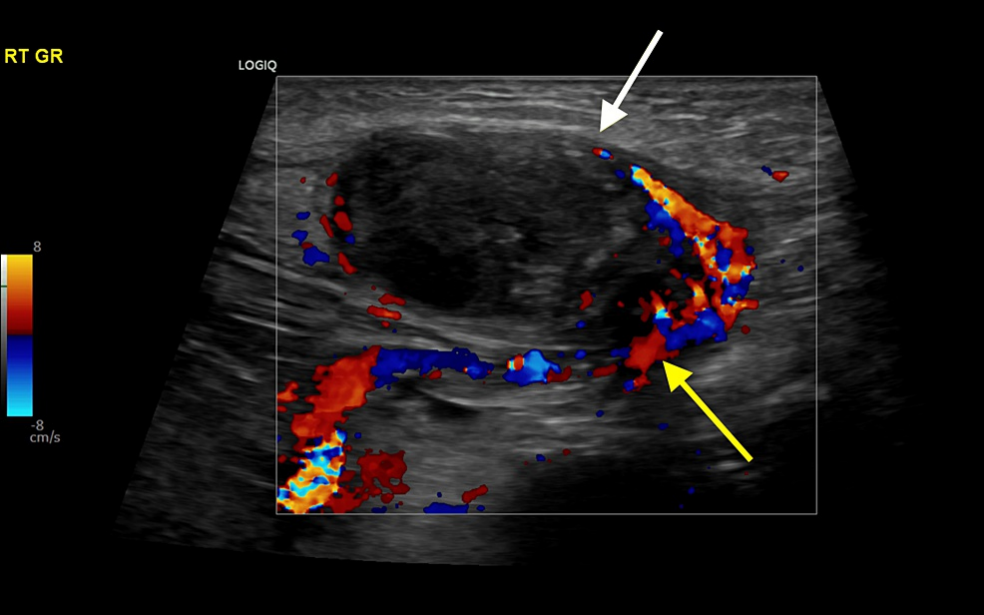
A color doppler ultrasound image of the right groin shows a heterogenous hypoechoic oval-shaped structure representing an infarcted testicle (white arrow), with peripheral vascularity representing a twisted spermatic cord (yellow arrow).
